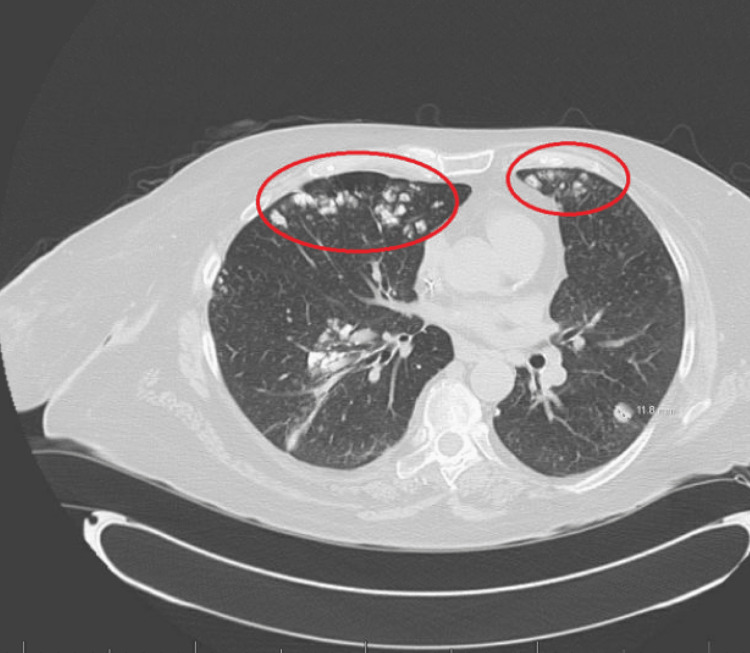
CT chest scan with contrast in October of 2020, showing bilateral anterior and apical pulmonary nodules and ground glass opacities.
